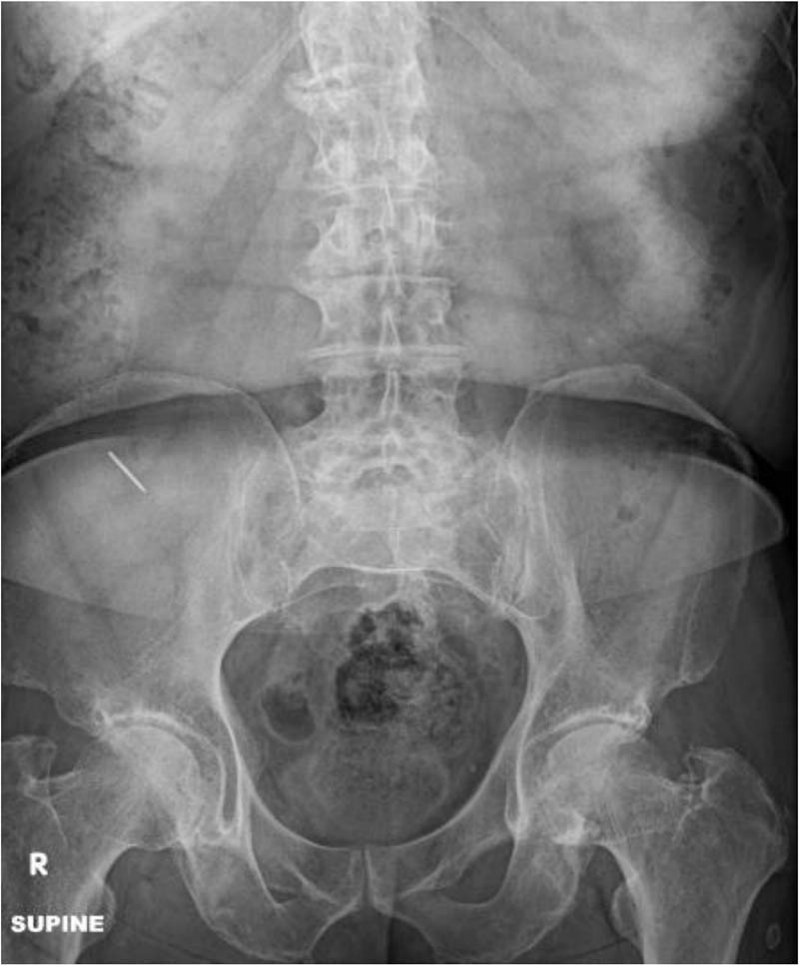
Abdominal radiograph shows a 2.3 cm linear radiopaque density in the right lower quadrant.
